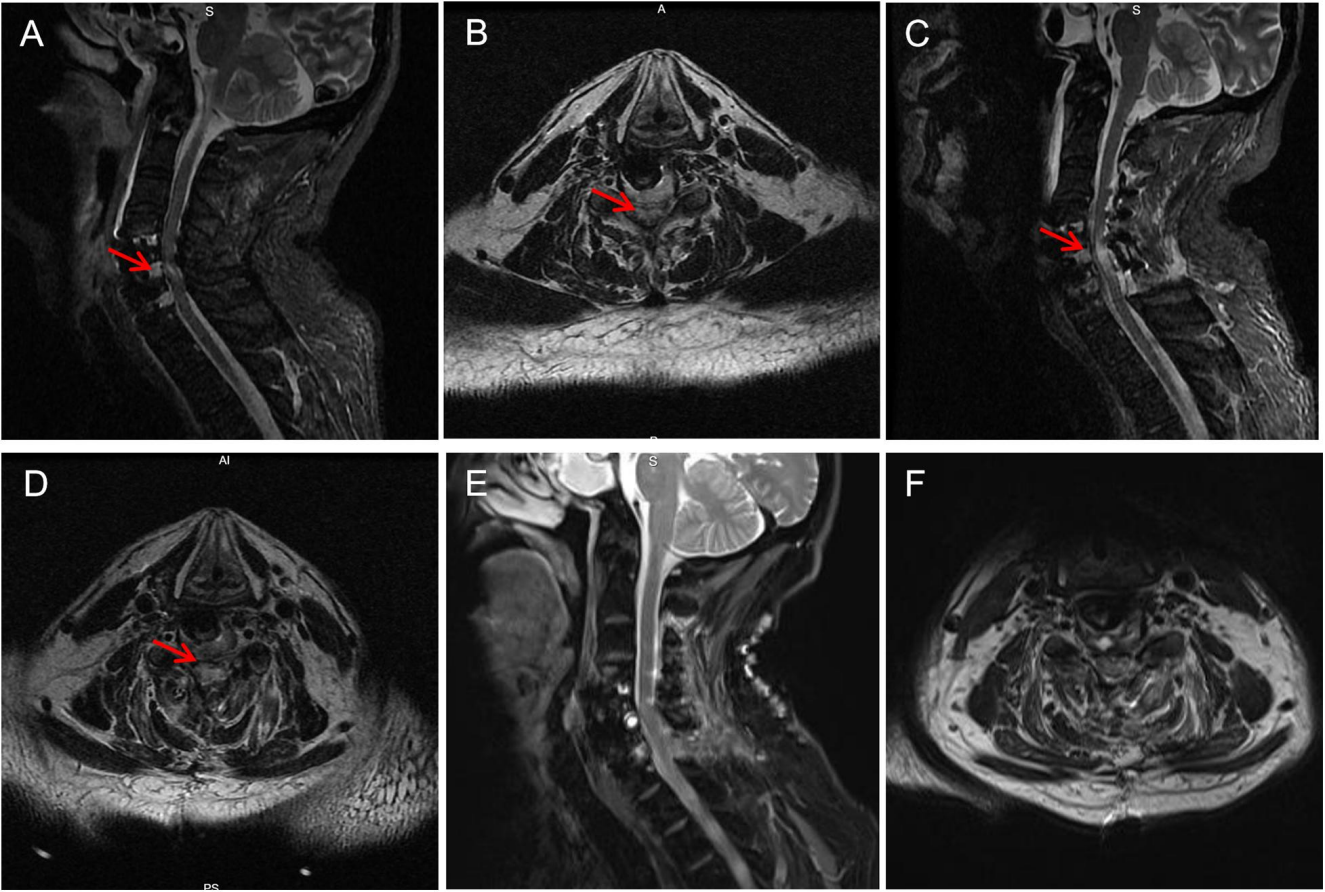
The patient underwent follow-up imaging examination after cervical spine surgery. (A) The sagittal plane of MRI shows high signal in the spinal cord part of C5-6,The physiological curvature of the cervical vertebrae is overextended; (B) the aqueous plane of MRI shows high signal in the spinal cord part of C5-6; (C) the sagittal plane of MRI shows a large amount of high signal in the C5-6 spinal cord, and the physiological curvature of the cervical vertebrae has partially recovered; (D) the horizontal MRI plane shows a large area of high signal in the C5-6 spinal cord; (E) the sagittal plane of MRI shows that the high signal of C5-6 spinal cord disappears; (F) the abnormal signal of the horizontal spinal cord in the C5surgical segment disappeared.
